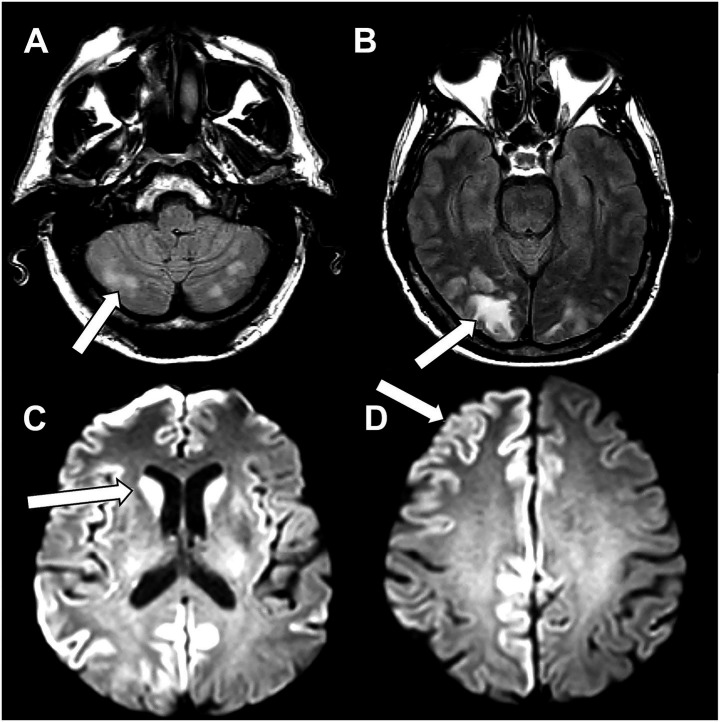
MRI findings demonstrating superimposed posterior reversible encephalopathy syndrome (PRES) in sporadic Creutzfeldt–Jakob disease (CJD). Axial FLAIR MRI sequences (A,B) demonstrate interval development of bilateral cerebellar and occipital cortical–subcortical T2/FLAIR hyperintensities consistent with superimposed posterior reversible encephalopathy syndrome (PRES). Diffusion-weighted imaging (C,D) demonstrates persistent asymmetric cortical ribboning and bilateral basal ganglia diffusion restriction characteristic of sporadic CJD, remaining largely unchanged despite interval development of PRES-related abnormalities. The coexistence of persistent prion-related diffusion abnormalities and evolving vasogenic edema complicated radiographic interpretation during the patient’s clinical deterioration.
